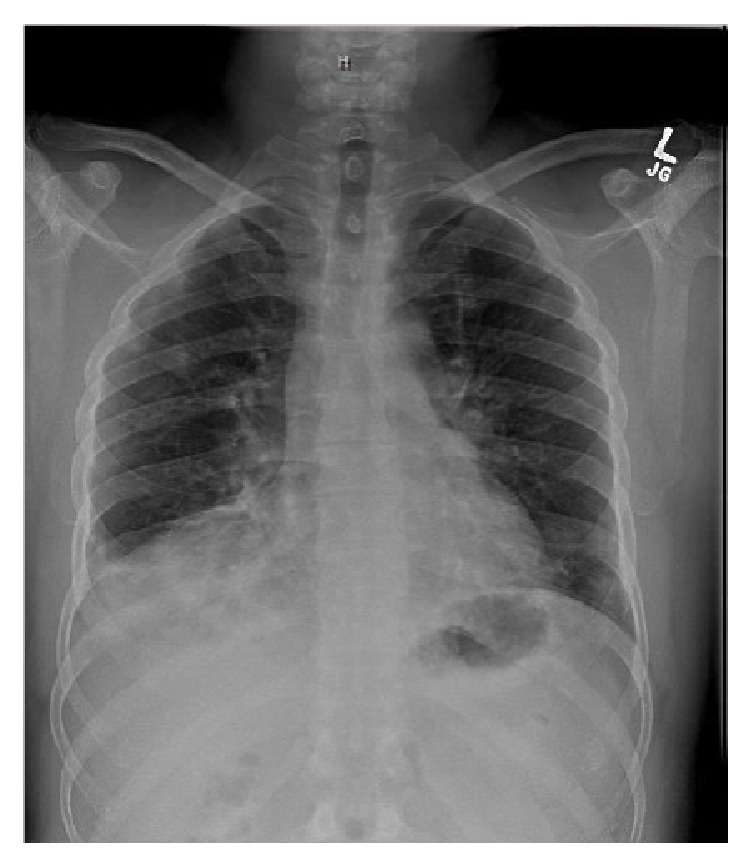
Chest X-ray showing bibasilar and right middle lobe infiltrate on presentation.
